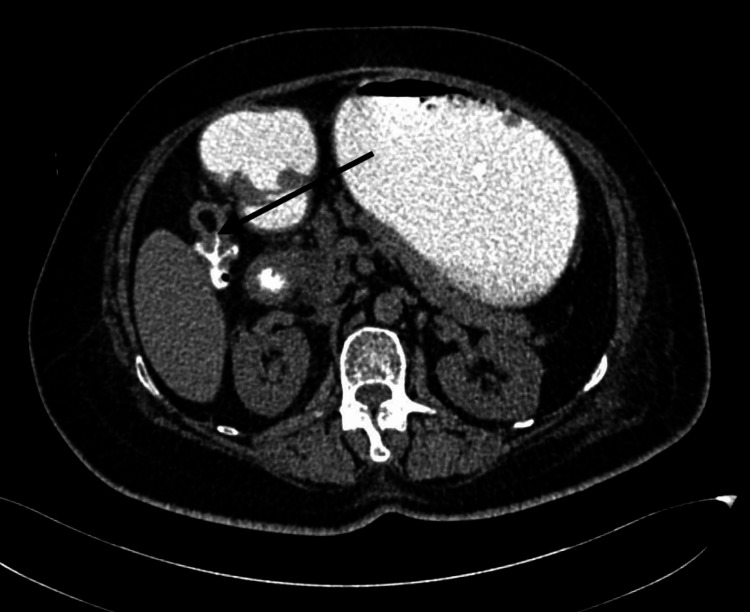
CT scan showing cholecystocolic fistula (black arrow)
